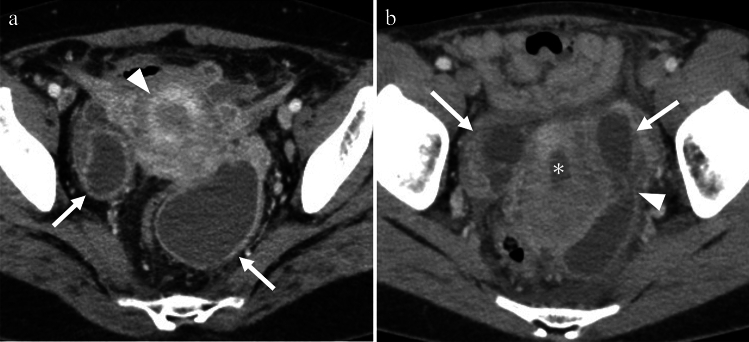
a Woman in her 40 s with fever and lower abdominal pain who tested positive for Chlamydia. Contrast-enhanced CT image demonstrates abnormal enhancement of the endometrium and hyperemia of the inner myometrium (arrowhead), indicating endometritis. Moreover, fluid collections with thick enhancing walls are visualized in both adnexas (arrows), suggesting tubo-ovarian abscesses. b Woman in her 40 s with fever and lower abdominal pain, positive for Gram-positive and Gram-negative rods The presence of complex fluid causing distention of the uterine cavity (asterisk) on contrast-enhanced CT is indicative of uterine empyema. In addition, the accumulation of fluid with thick enhancing walls in both adnexa (arrows) suggests the presence of tubo-ovarian abscesses. Furthermore, there is evidence that the left TOA has perforated the abdominal cavity (arrowhead). A fatty haziness of the parametrium is also observed.
